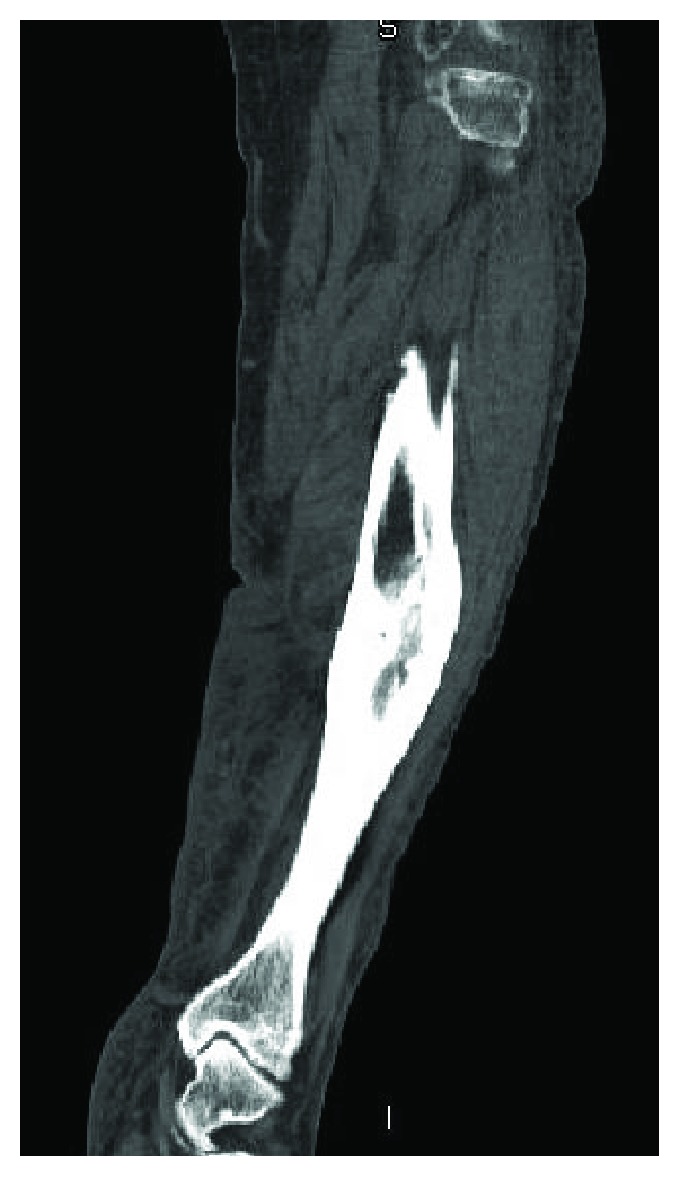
CT scan—malunited fracture of the middle third left tibia.
